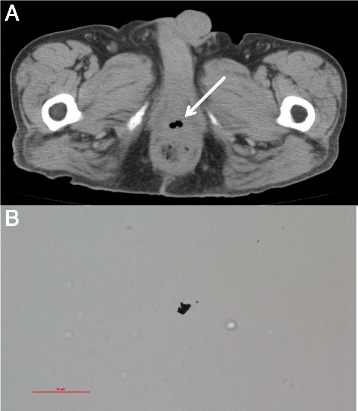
a Case 1. CT scan revealed the presence of a small amount of extraluminal air between the prostate and rectum, adjacent to the coloanal anastomosis (*arrow*). b Case 1. Orally administered medicinal charcoal was detected in the urine
